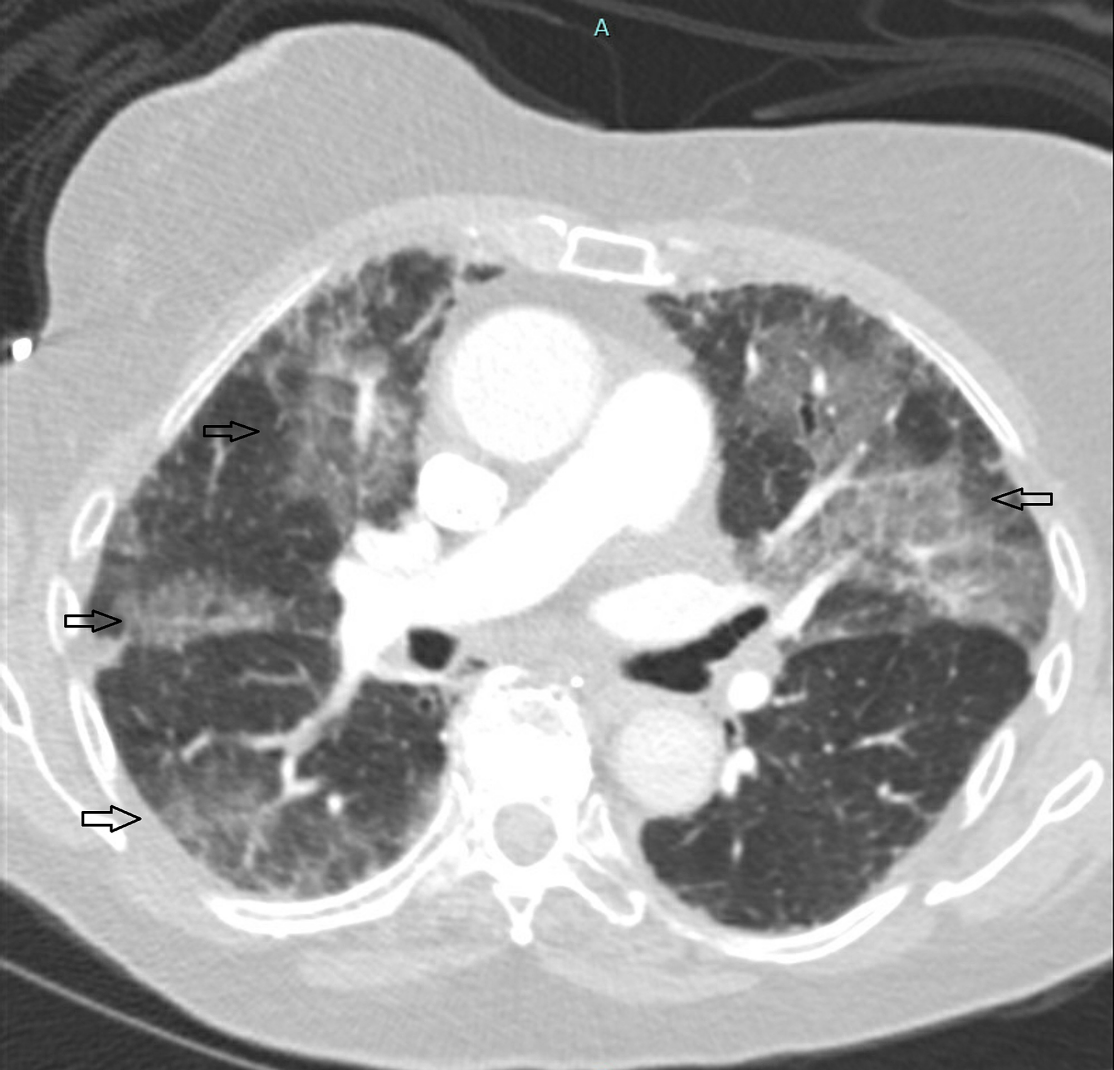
Contrast CT scan chest. Arrows showing patchy areas of ground glass opacity
